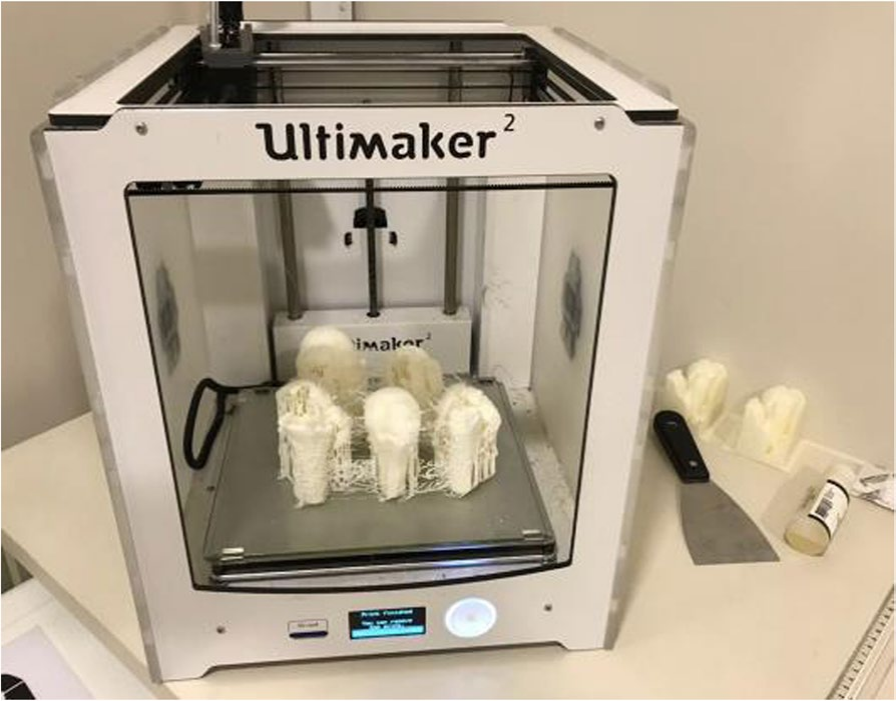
3D printing models of proximal humerus fractures using CT scans as a model
